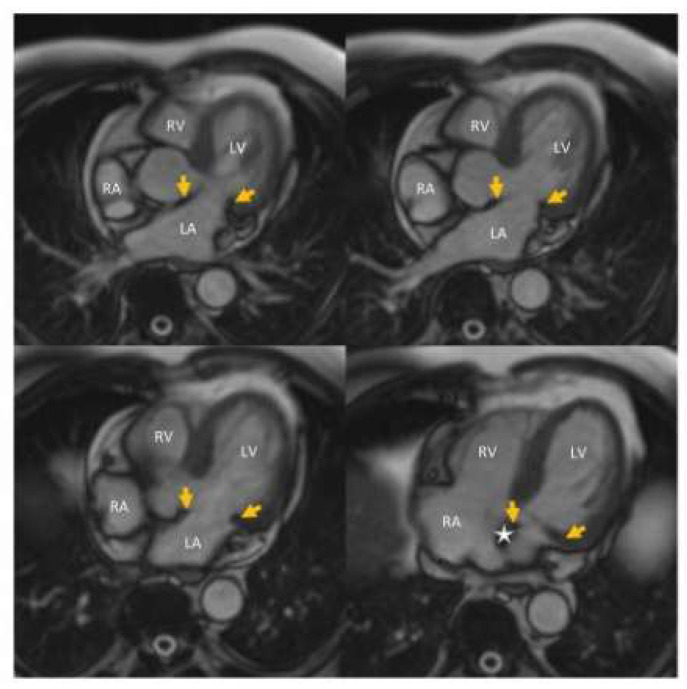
Cine movie cardiac MR images in transversal planes. Arrows show the mitral annular calcification. Suspected area of the AV node is indicated by asterisk, showing its proximity to MAC.
